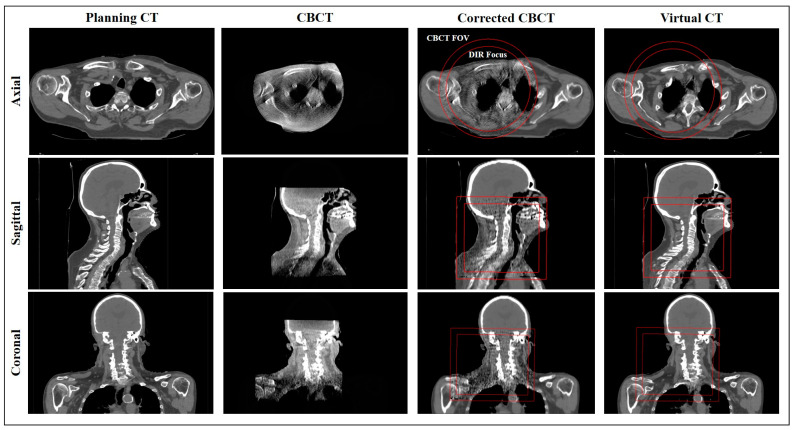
A patient example showing axial, sagittal, and coronal views for the pCT, CBCT, corrCBCT, and virtCT. Two ROIs are demonstrated on the axial view of the corrected CBCT: the field of view ROI and the field of view ROI retracted 2 cm, which is used to focus the deformable image registration.
